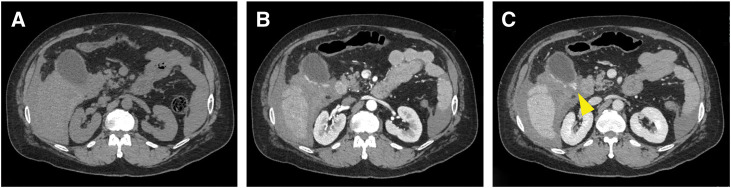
Dynamic CT findings. (A) Plain CT. (B) Arterial phase showing early enhancement on the dorsal aspect of the gallbladder. (C) Parenchymal phase showing extravasation from the same area (yellow arrow).
